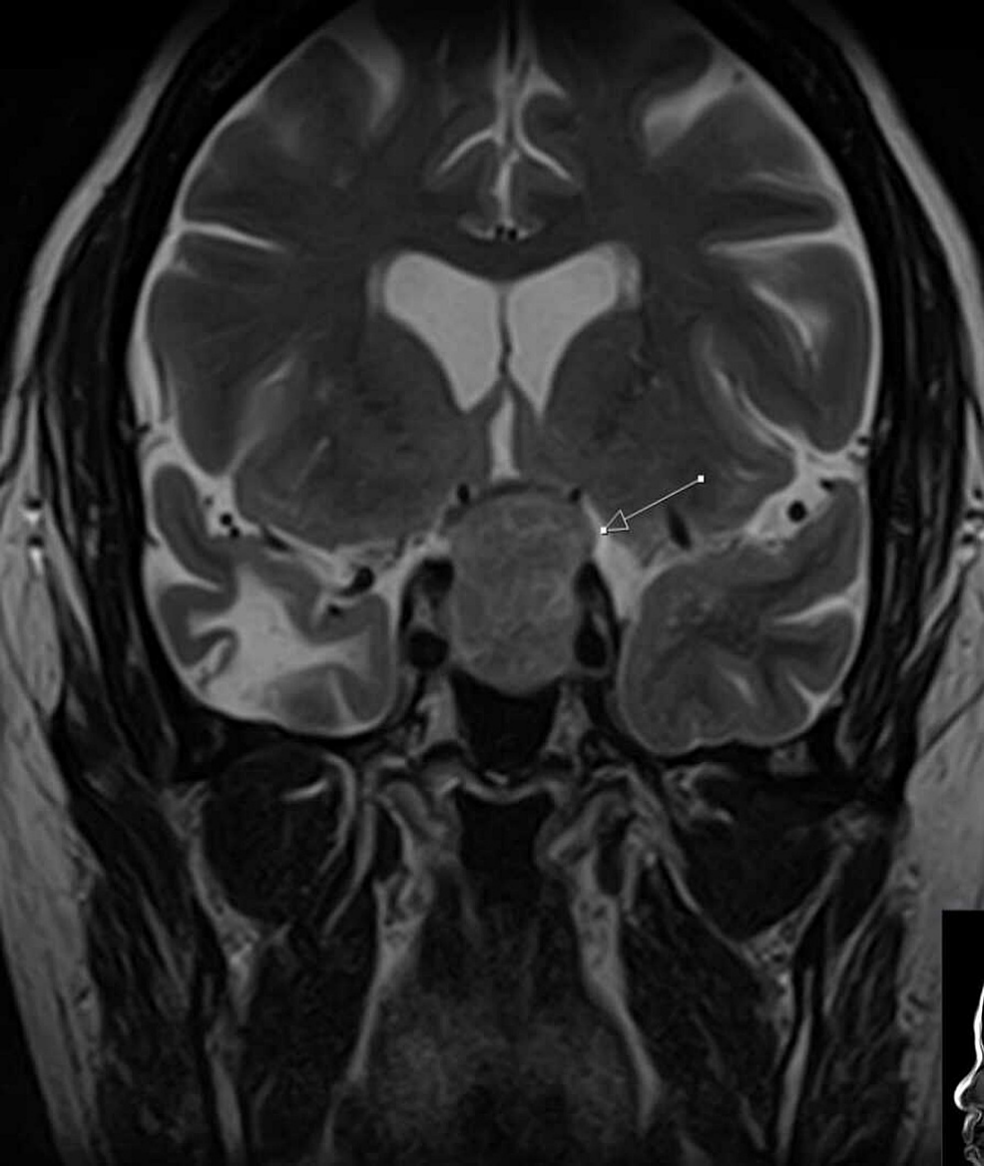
MRI shows pituitary tumor
